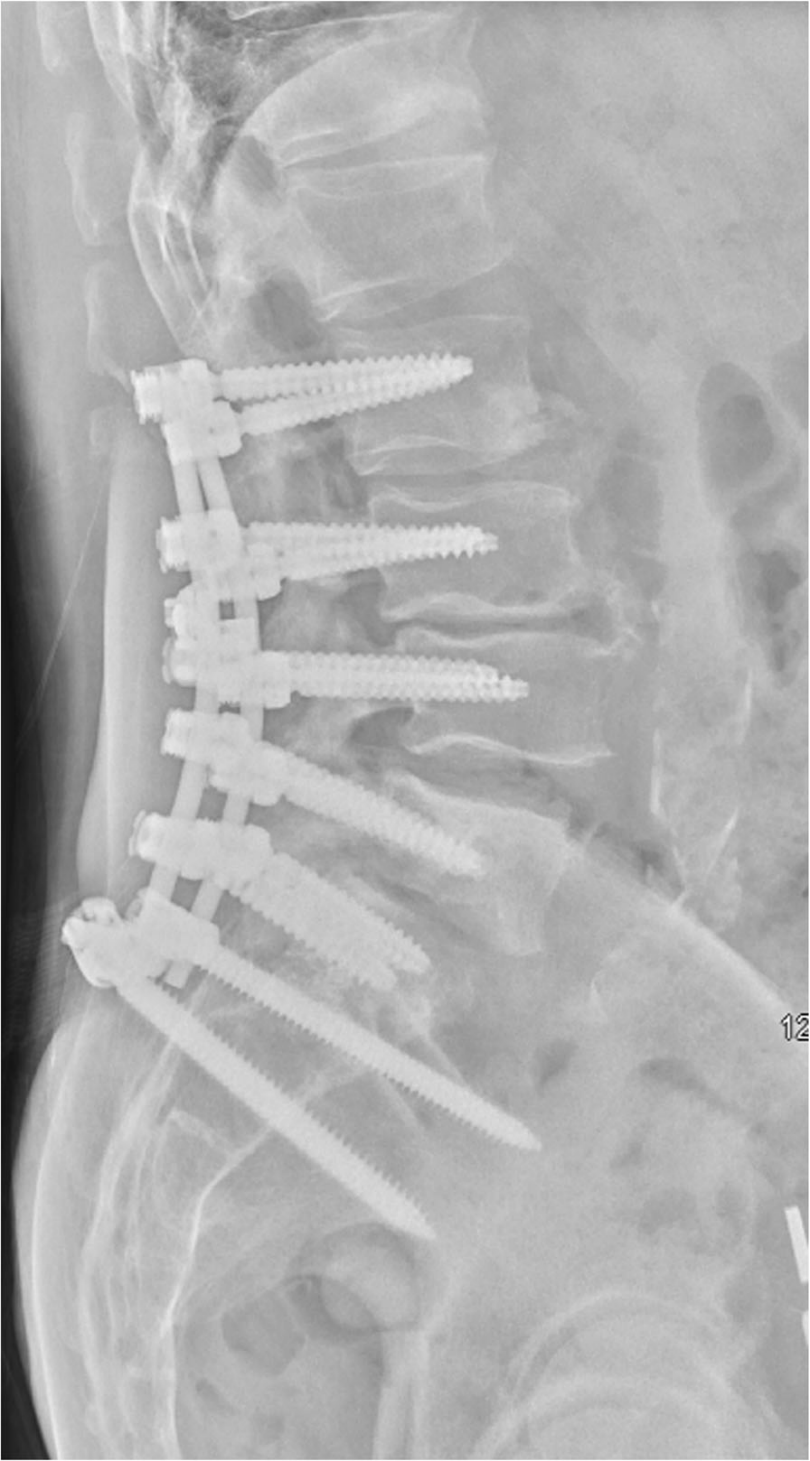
Lateral Lumbar X-ray: L2-Pelvis fusion construct with maintenance of lordotic alignment
